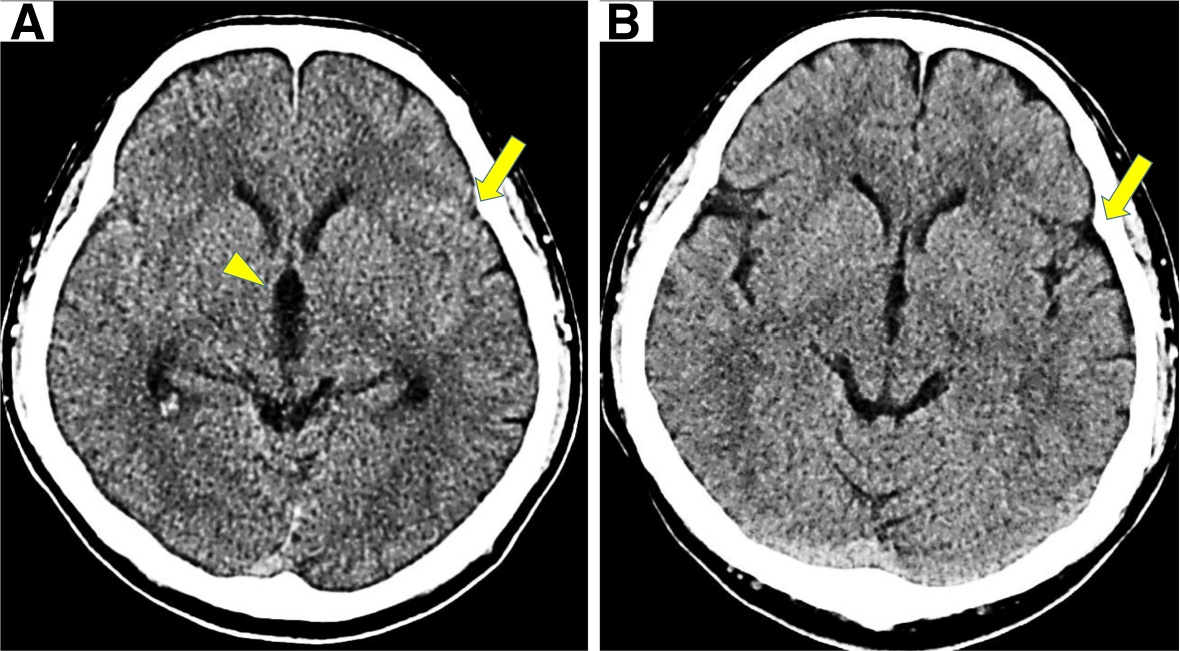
Computed tomography (CT) image of the current patient showing Sylvian fissures, which were not clearly visible bilaterally (a), compared to a CT image of the same patient taken 2 years ago when the patient complained of occipital headache, nausea, and vomiting. At that time, no abnormalities were detected (b). There was mainly isoattenuating SAH in the Sylvian fissures (slightly hyperattenuating on the left) and mild hydrocephalus - seen as enlargement of the third ventricle (a) in comparison to the previous CT (b). An *arrow* indicates Sylvian fissure, and an arrowhead indicates the dilated third ventricle
